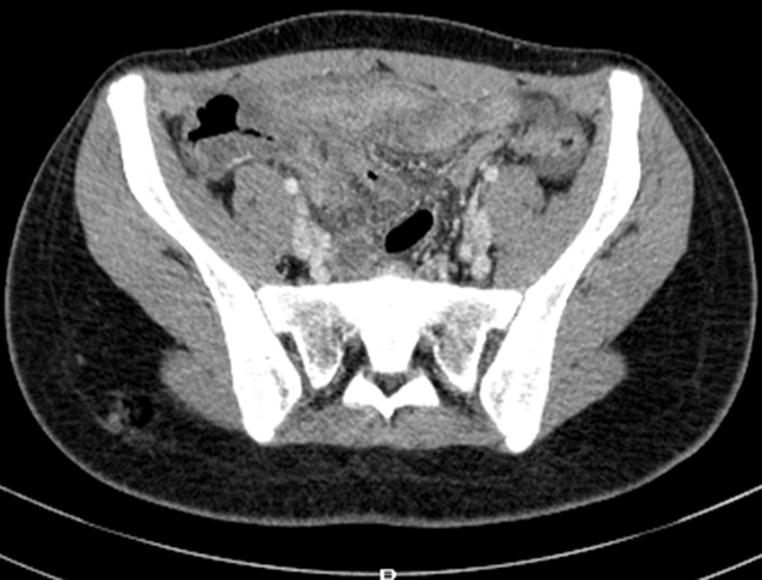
an axial CT scan of abdomen showing inflammatory features surrounding a blind-ending structure arising from the caecum
